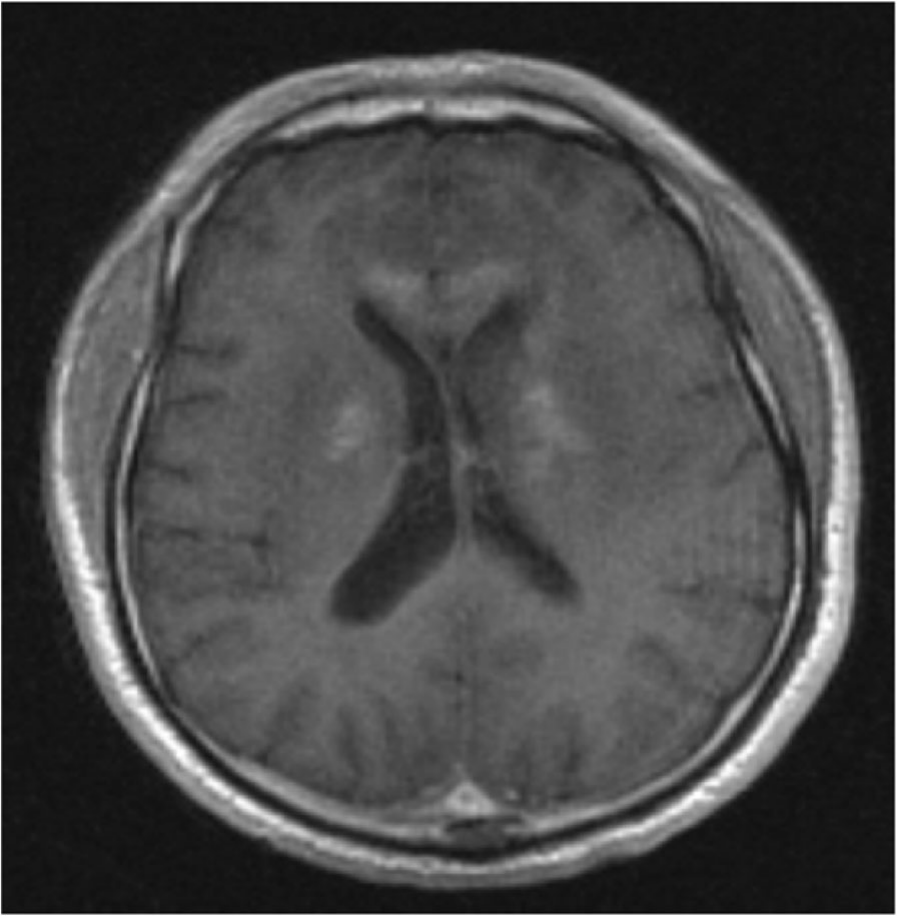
Magnetic resonance imaging shows bilateral enhancement involving corona radiata extending toward basal ganglia
